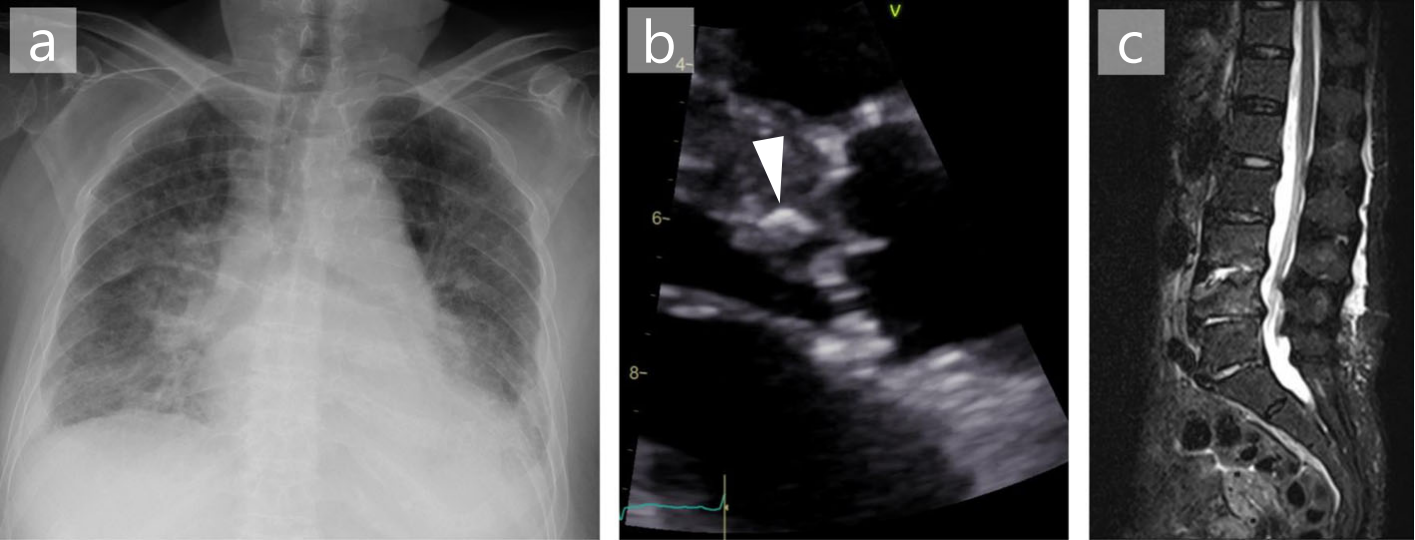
Diagnostic imaging of *Enterococcus casseliflavus* endocarditis. a. Chest radiograph showing bilateral interstitial opacities, pleural effusion, and slight cardiac dilatation; b. Transthoracic echocardiogram (parasternal long-axis view) showing aortic valve with vegetation (arrowhead); c. A lumbar MRI STIR image showing osteolytic lesion at the L3/4 level
